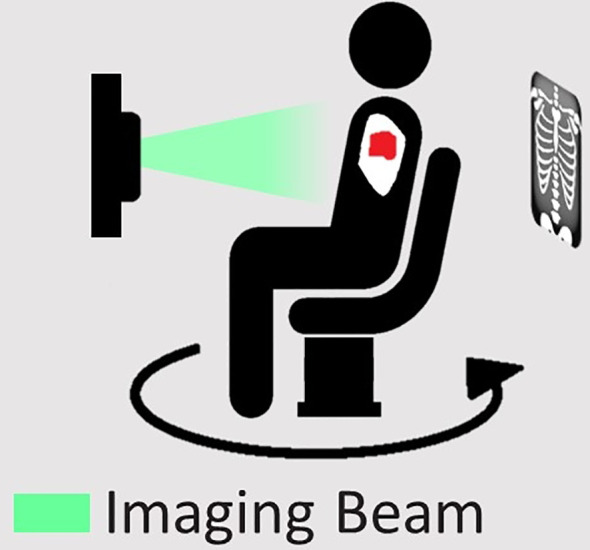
• High-quality 3D and 4D images of an upright patient • Accurate tissue classification/HU for dose calculation • Geometrically accurate	• Upright CT/MRI scanner • Linac onboard CBCT with HU corrections • Deformable registration from recumbent multi-modality imaging
